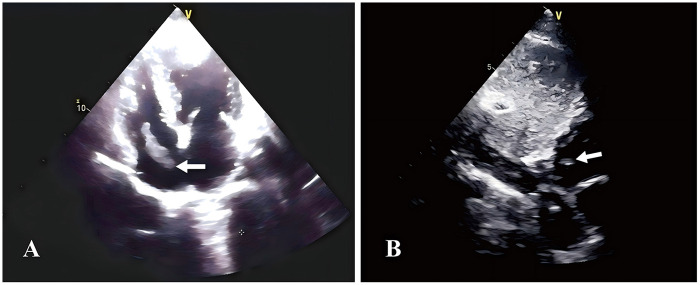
Transthoracic echocardiographic images of intravenous leiomyomatosis with intracardiac extension. (A) Apical four-chamber view shows a hypoechoic tumor mass (arrows) in the right atrium of Patient 3. (B) Subcostal view demonstrates tumors (arrows) in the right atrium of Patient 5.
